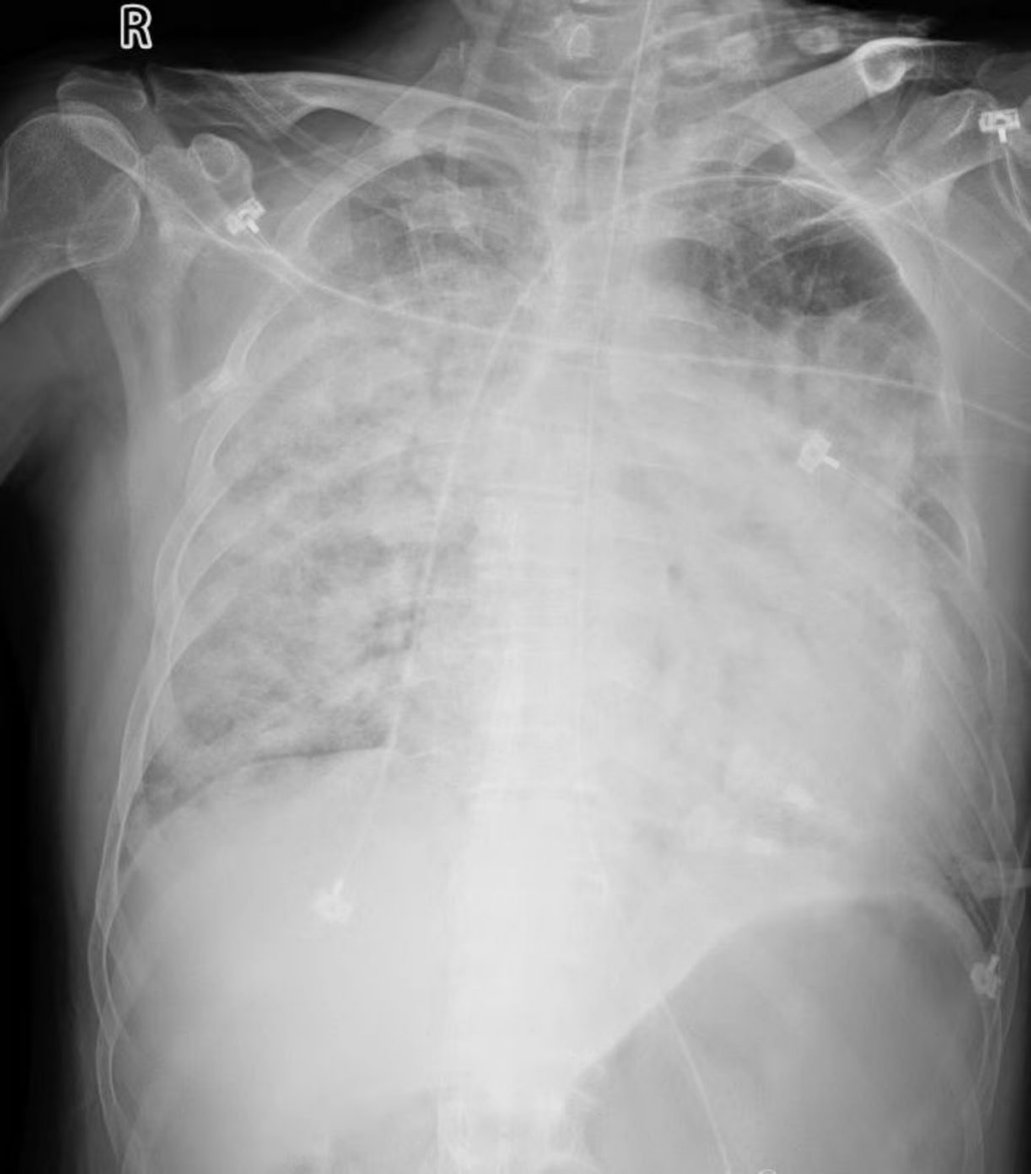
Chest X-ray of the patient after effective anti-infective treatment.
